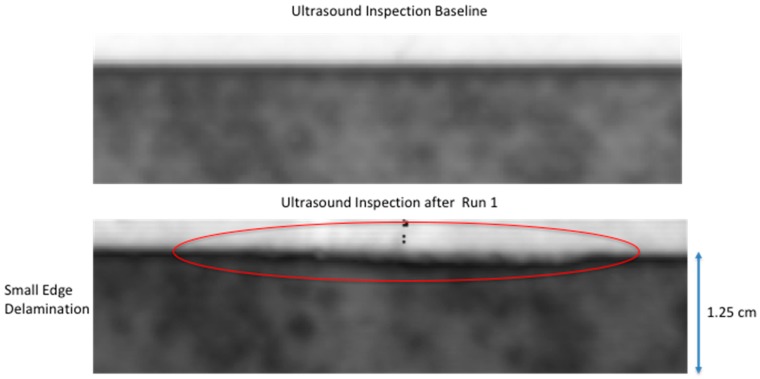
Ultrasonic inspection image after initial quasi-static load run with comparison to baseline ultrasonic inspection.
